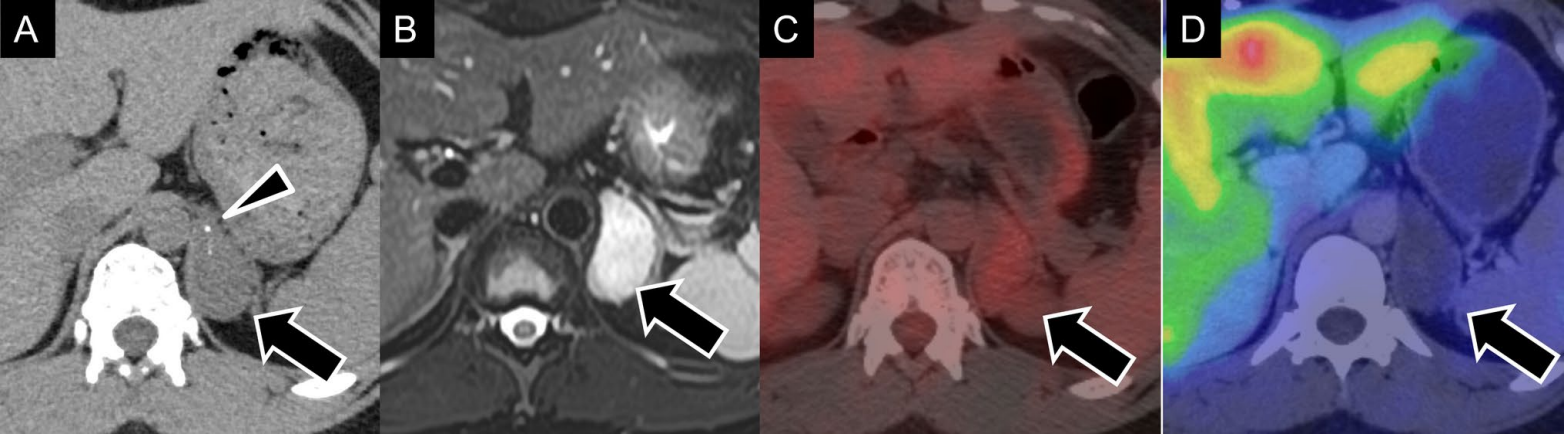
Imaging findings of the ganglioneuroma. A 31-year-old female with an incidentaloma. A Unenhanced computed tomography (CT) showing a well-circumscribed mass in the left = adrenal gland (arrow) with fine speckled calcifications (arrowhead). B T2-weighted magnetic resonance imaging showing a high signal intensity of the mass (arrow). C Fusion single-photon-emission computed tomography/computed tomography images showing mild ^123^I-metaiodobenzylguanidine uptake in the lesion (arrow), which can occasionally be seen in ganglioneuroma, although negative scans are more typical. D Fusion positron-emission tomography/computed tomography images showing no significant ^1^⁸F-fluorodeoxyglucose (^1^⁸FDG) uptake within the tumor (arrow), consistent with ganglioneuroma. Pathology confirmed the diagnosis of ganglioneuroma
